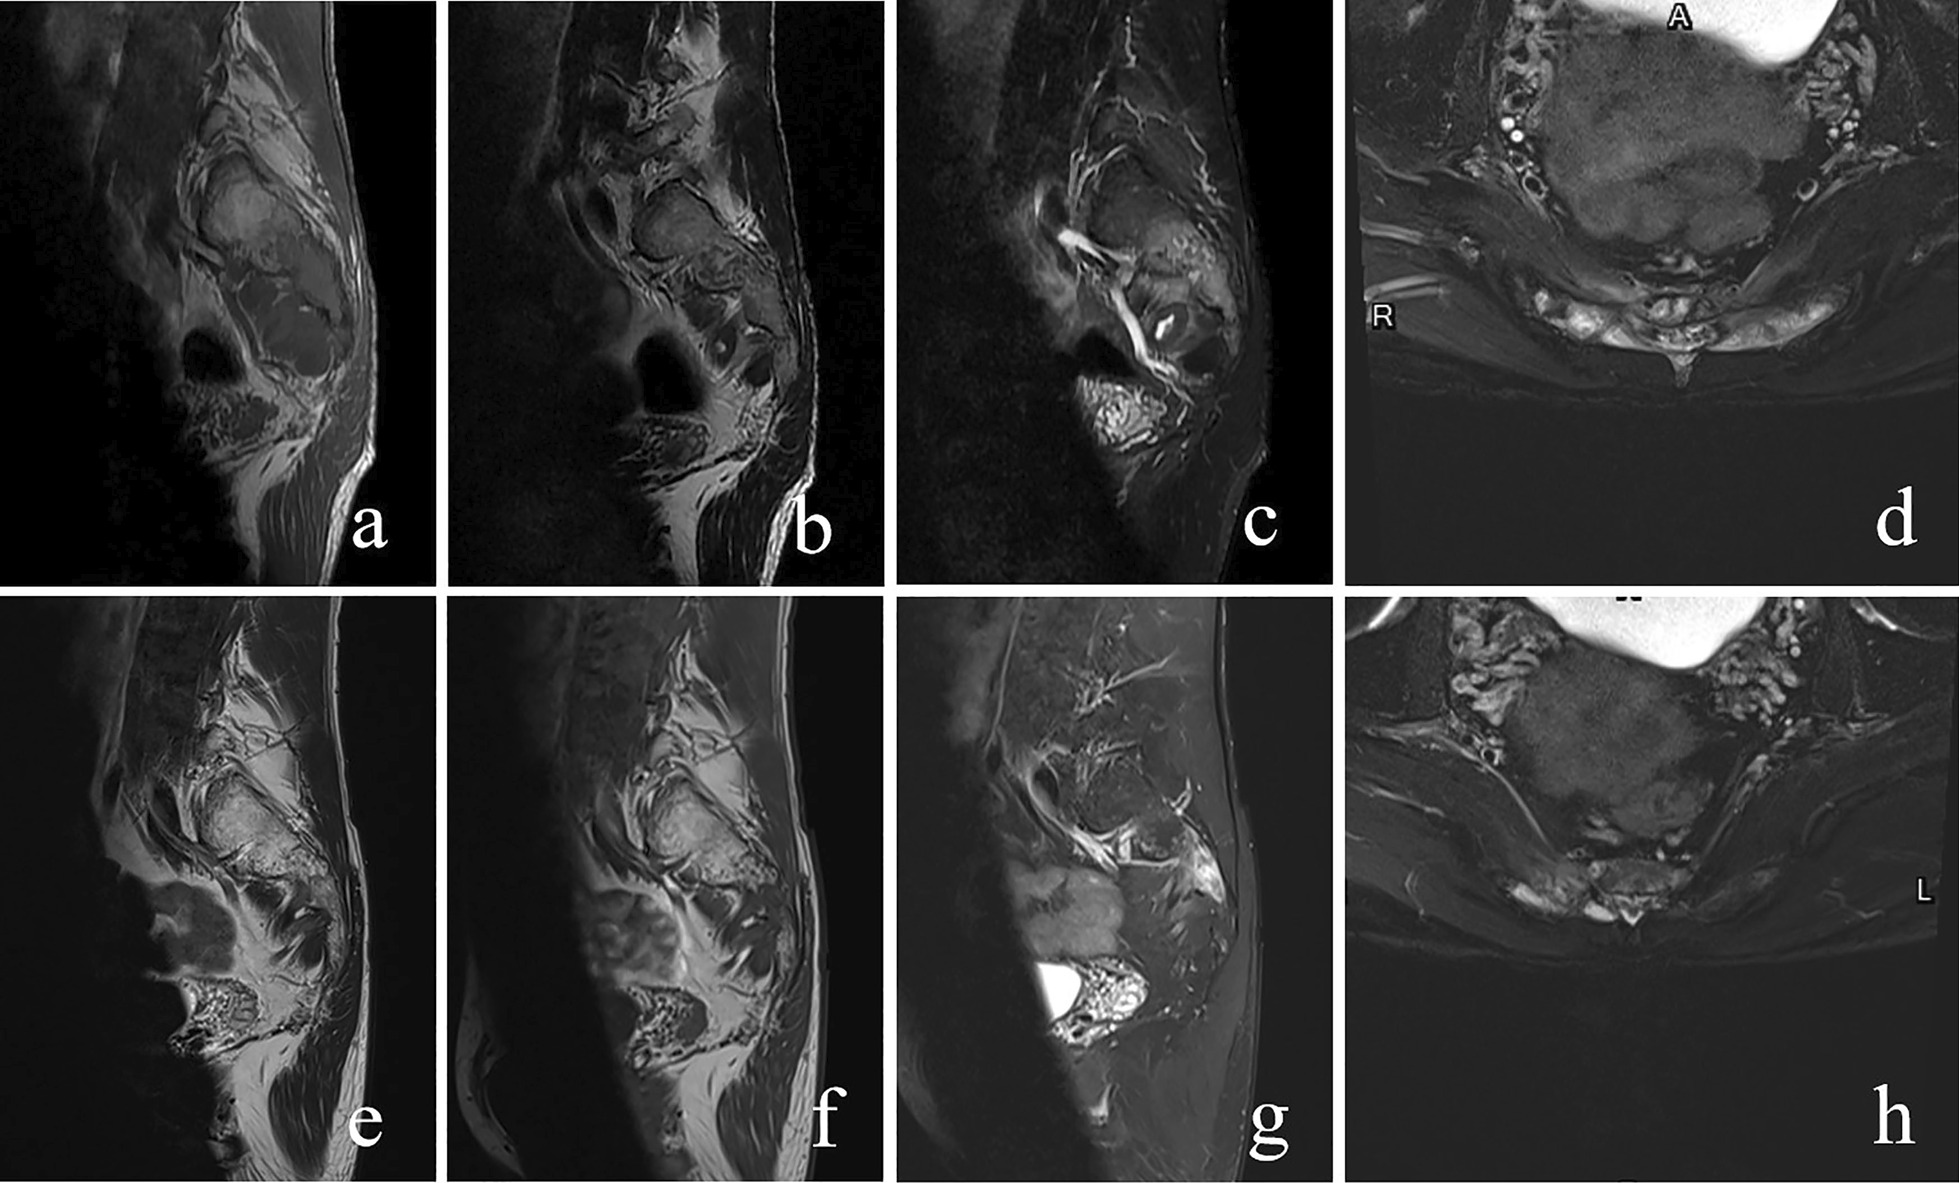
Postoperative magnetic resonance imaging (MRI)(sacrum). One-month post-operative MRI (a sagittal T1-weighted; b sagittal T2-weighted; c sagittal fat-suppressed T2-weighted; d transversal fat-suppressed T2-weighted ): abscess became smaller. Six-month post-operative MRI (e sagittal T1-weighted; f sagittal T2-weighted; g sagittal fat-suppressed T2-weighted; h transversal fat-suppressed T2-weighted ): abscess resolved
